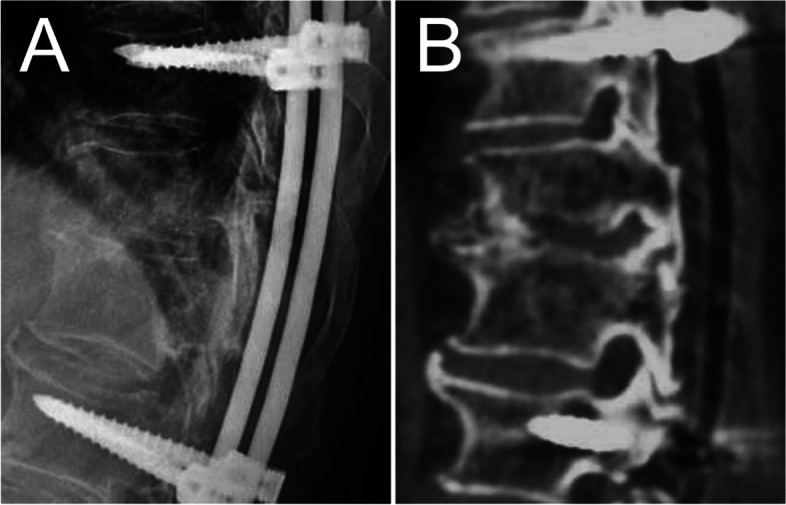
A 72-year-old male 6 months after surgery. A Sagittal X-ray showed indistinct bone connection between the affected segments and B Sagittal CT reconstruction showed obvious peripheral bone bridge formation
